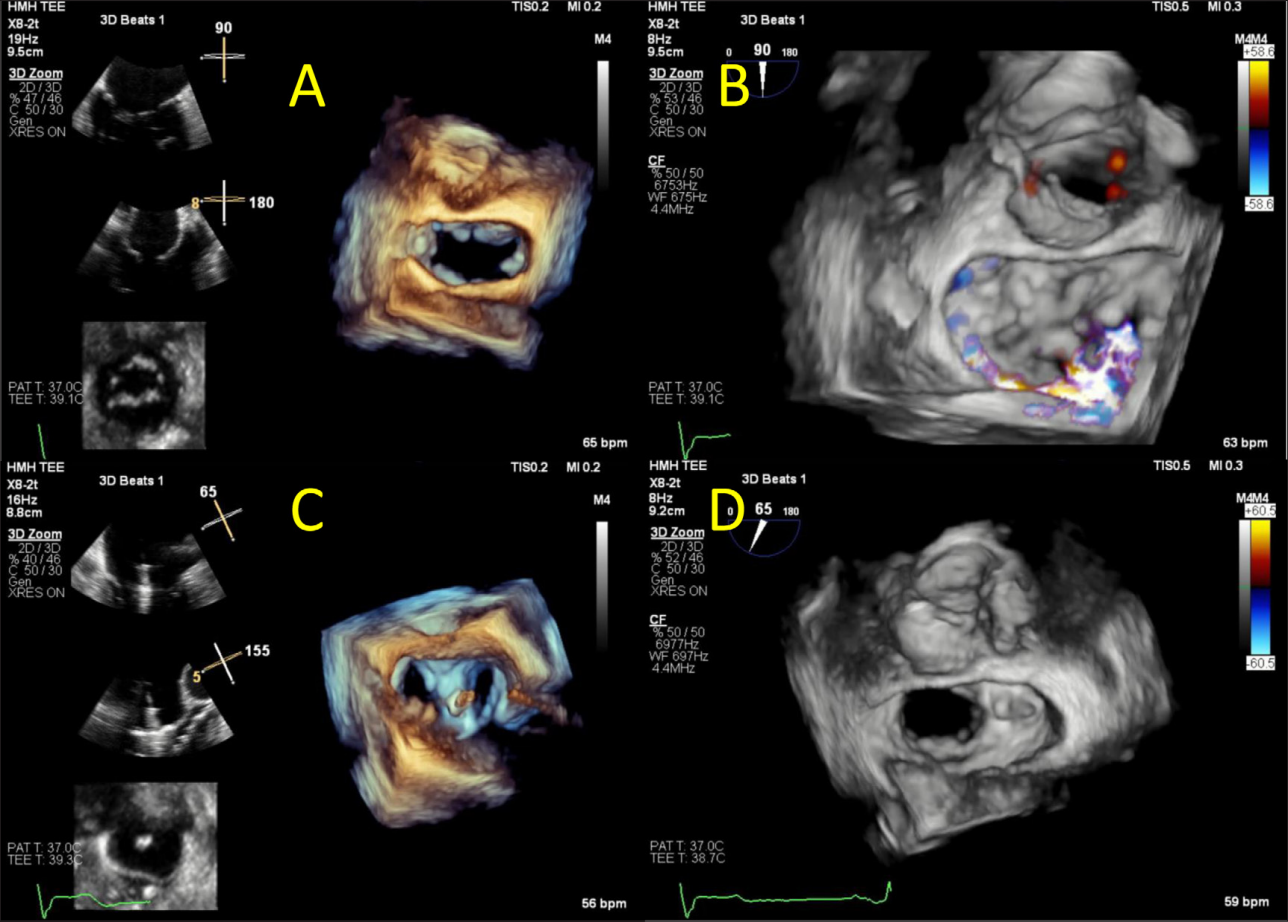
Transesophageal echocardiogram showing (A) 2D and 3D video clip of the mitral valve showing medial aspect of A2 and A3 prolapse. (B) 3D color Doppler video clip of the mitral valve showing posteriorly directed mitral regurgitation. (C) 2D and 3D video clip of the mitral valve showing the guide catheter and MitraClip position after grasping A2 and P2 scallops. (D) 3D color Doppler video clip of the mitral valve after deploying the MitraClip. Mild residual MR noted. See Figure 4 A videoclip at https://youtu.be/NRvFQL6UBJA, Figure 4 B videoclip at https://youtu.be/wTsxav-JLbo, Figure 4 C videoclip at https://youtu.be/xH8RG0NrHp4 and Fig4D videoclip at https://youtu.be/yMQjrnIhM_0.
